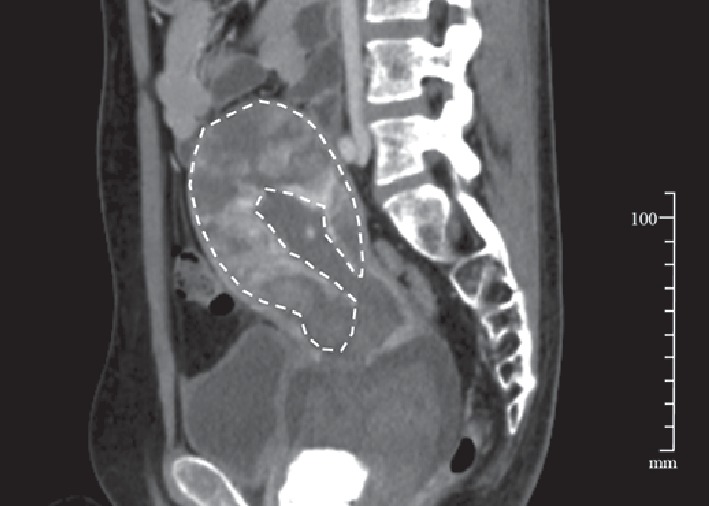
Sagittal contrast-enhanced computed tomographic image of the uterus on the 10^th^ postpartum day. The retained placenta is circumscribed by a dashed line. Note that the placenta is enhanced by contrast medium in a patchy manner.
